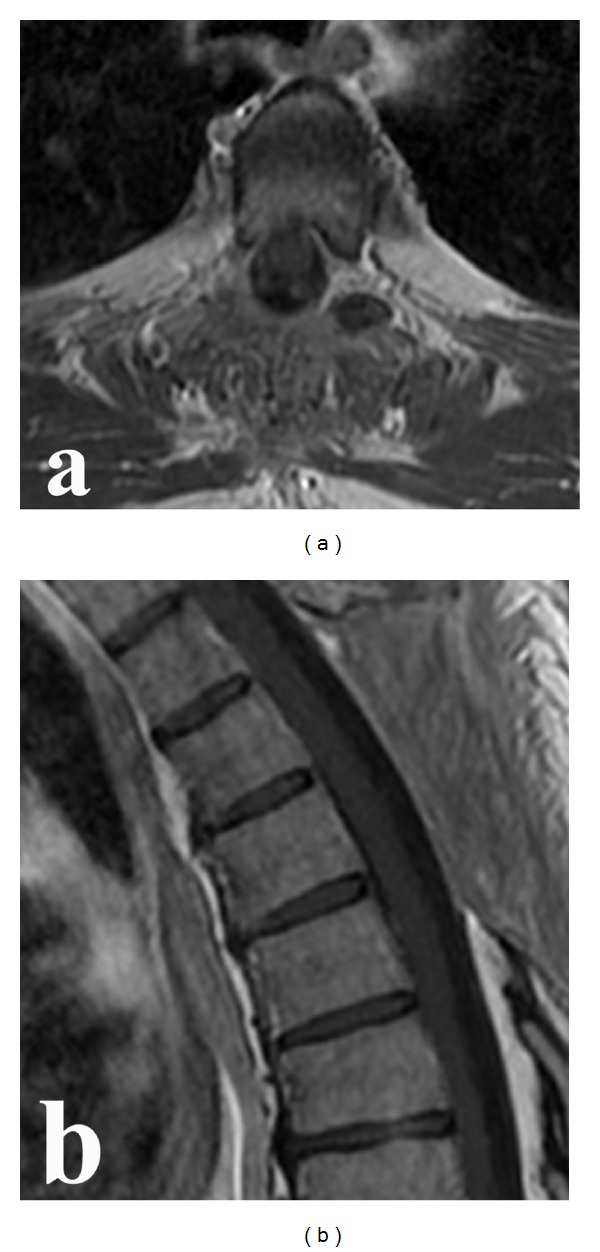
Followup axial (a) and sagittal (b) T1-weighted magnetic resonance imaging. Postoperative contrast enhanced magnetic resonance imaging scans obtained one year after the operation, local radiotherapy, and chemotherapy demonstrated no evidence of relapse.
